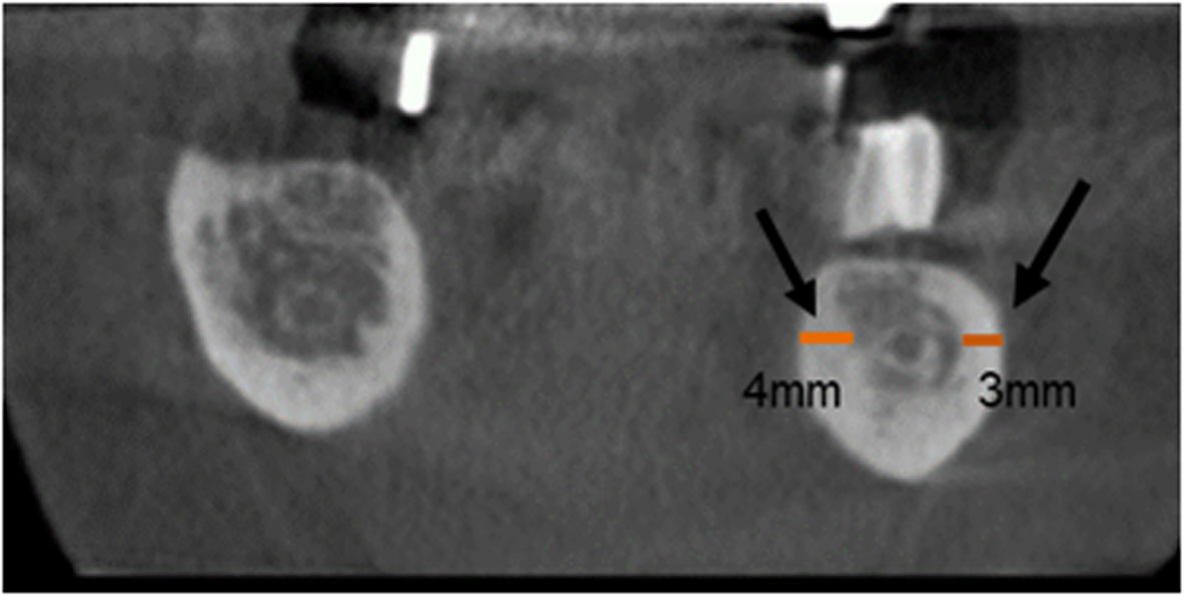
Section of CBCT image of region 36 with buccal and lingual measurement of cortical bone thickness
